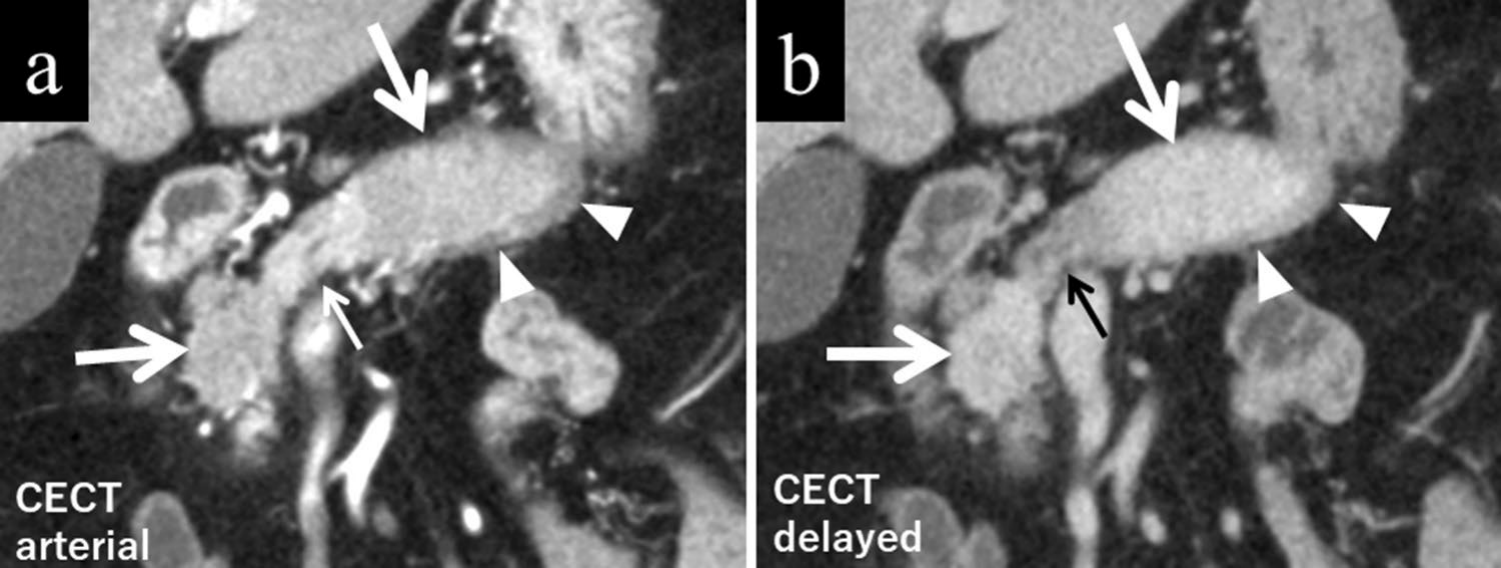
A male patient in his 70 s with typical autoimmune pancreatitis (AIP). a There is localized sausage-like swelling of the pancreas affected by AIP (large arrows). These lesions are hypodense during the pancreatic phase as compared to the normal pancreas (small arrow). Typical rim enhancement (arrowheads) demarcating the pancreatic tail is seen. b The same coronal section on delayed-phase CT reveals the lesion is hyperdense during the delayed phase compared to the normal pancreatic parenchyma (small arrow). The rim is also discernible in the delayed phase (arrowheads)
